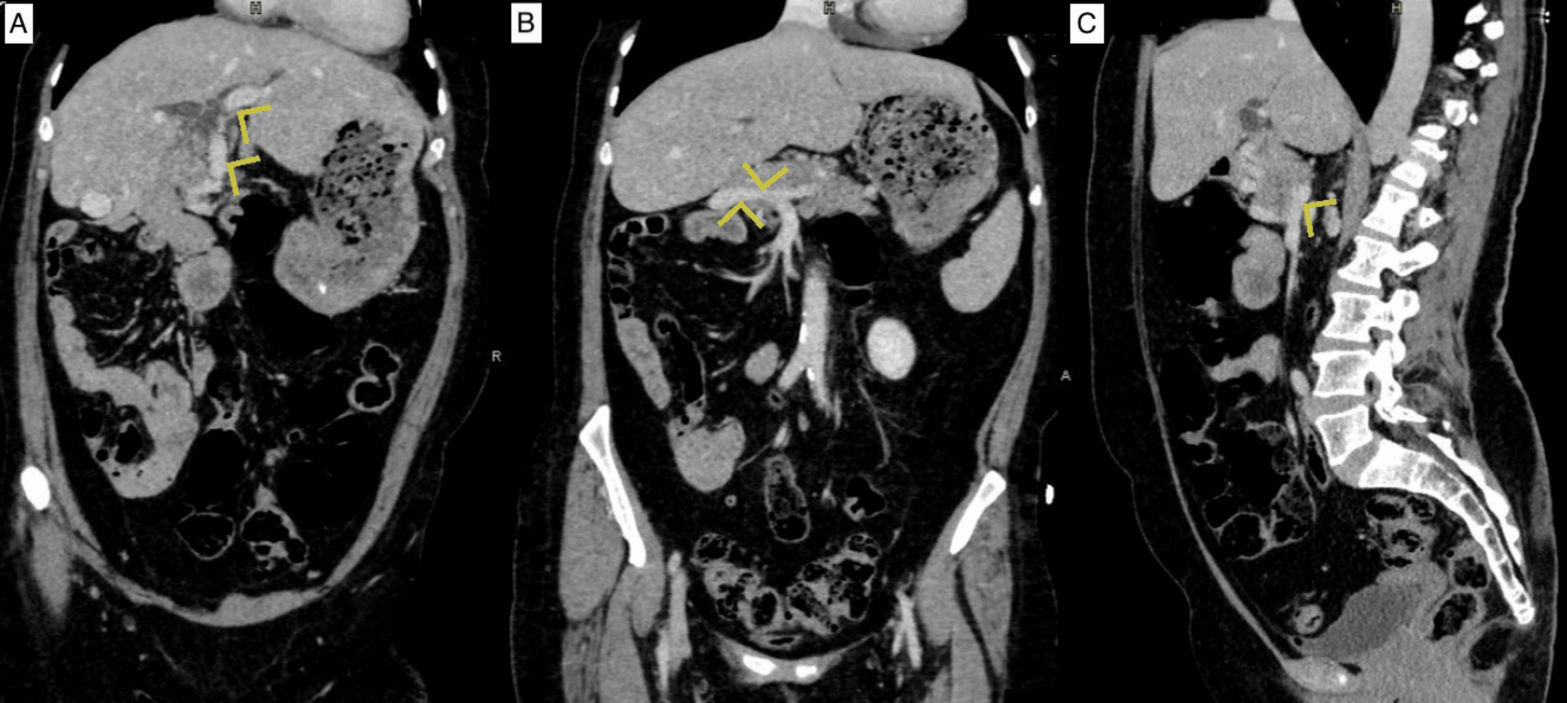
Computed tomography coronal and sagittal views showing the portal vein (yellow arrows) coursing anterior to the pancreas up to the porta hepatis (A), the second portal vein (yellow arrows) joined by the splenic vein posterior to the pancreas (B, C).
